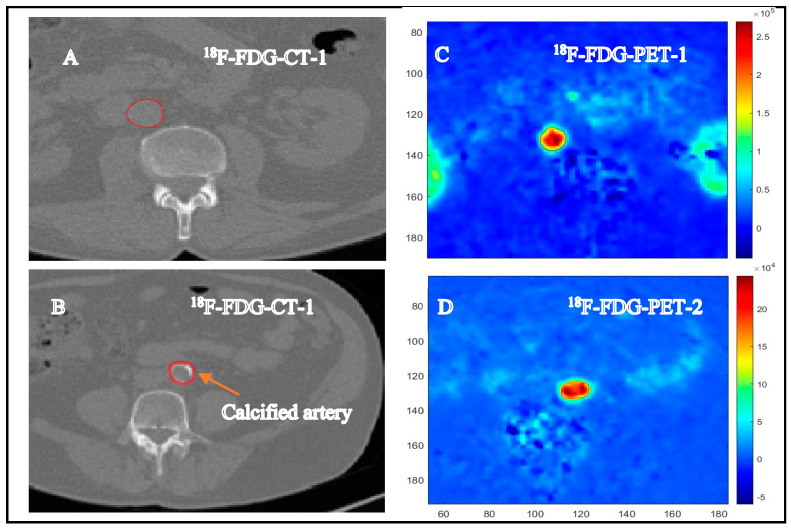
Transaxial PET/CT images of the aortas from one patient. Panels (A,C) portray the same arterial slice, which is devoid of CT-detected calcification. The PET scan in panel C detected significant ^18^F-FDG uptake. The corresponding CT and PET-^18^F-FDG of a calcified artery are, respectively, shown in (B,D). The arrow designates the calcified segment within the aorta.
